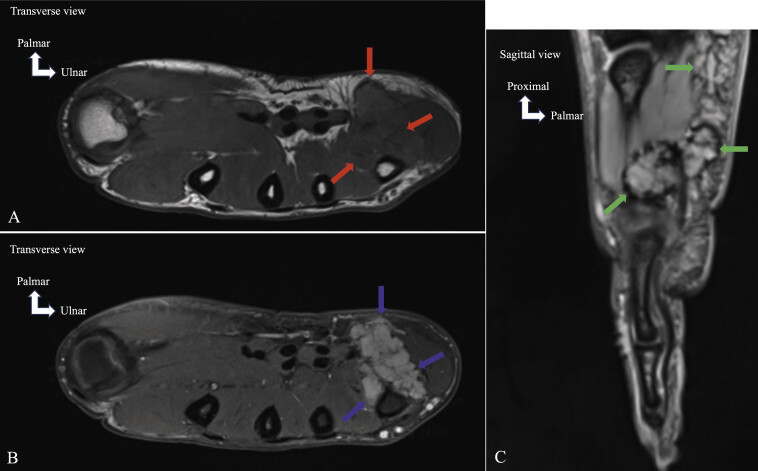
Tenosynovial giant cell tumor. MRI on axial T1-weighted (
